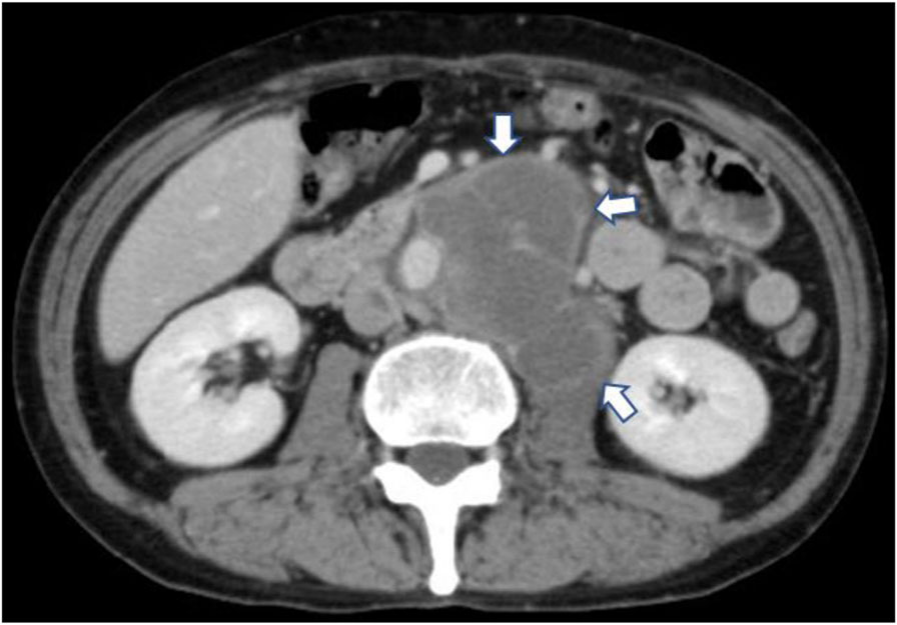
Enhanced computed tomography at initial examination showing the large mass lesion along with the abdominal aorta. The margin of the mass lesion is enhanced (arrow)
